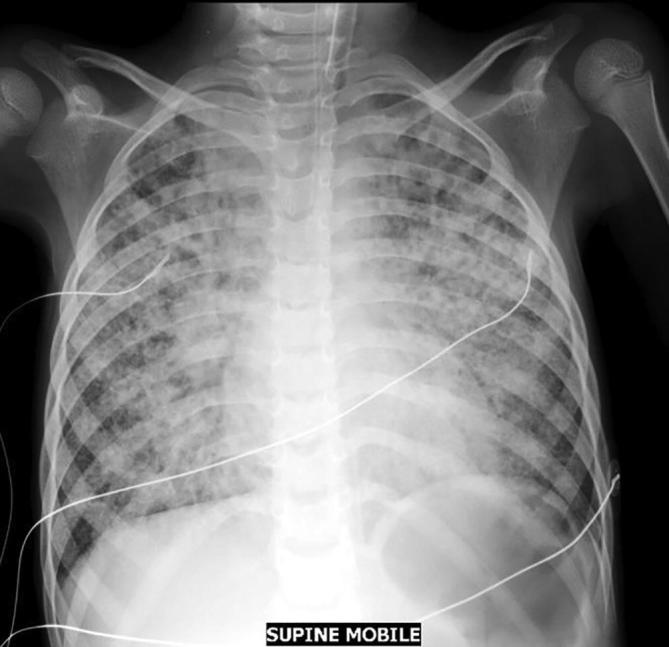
Plain chest radiograph image of a young child with pneumonia who cultured *M. tuberculosis* on an induced sputum sample.
